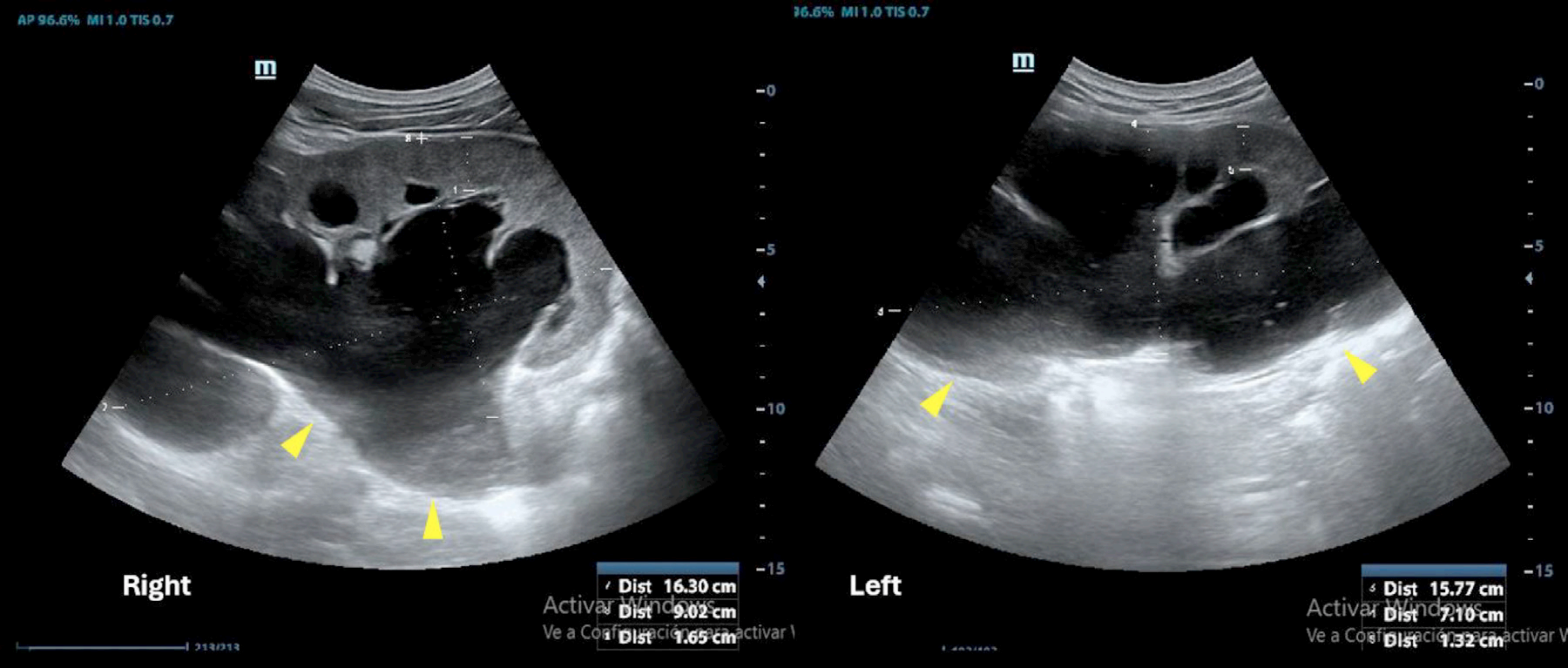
Renal ultrasound showed severe bilateral hydronephrosis.
